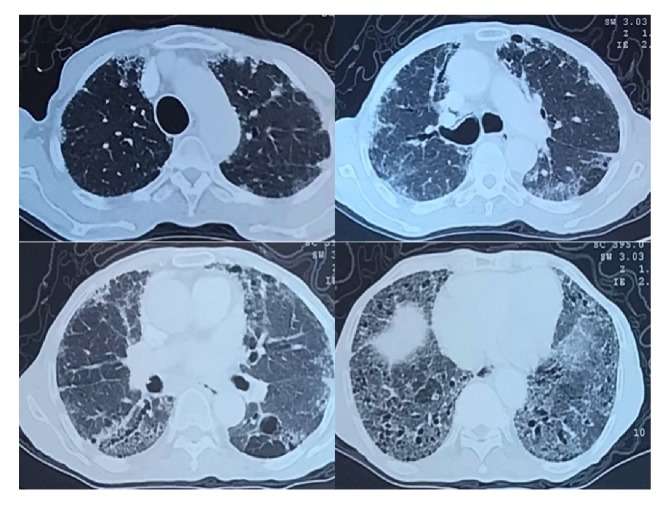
Control CT scan showing regression of the pneumomediastinum.
